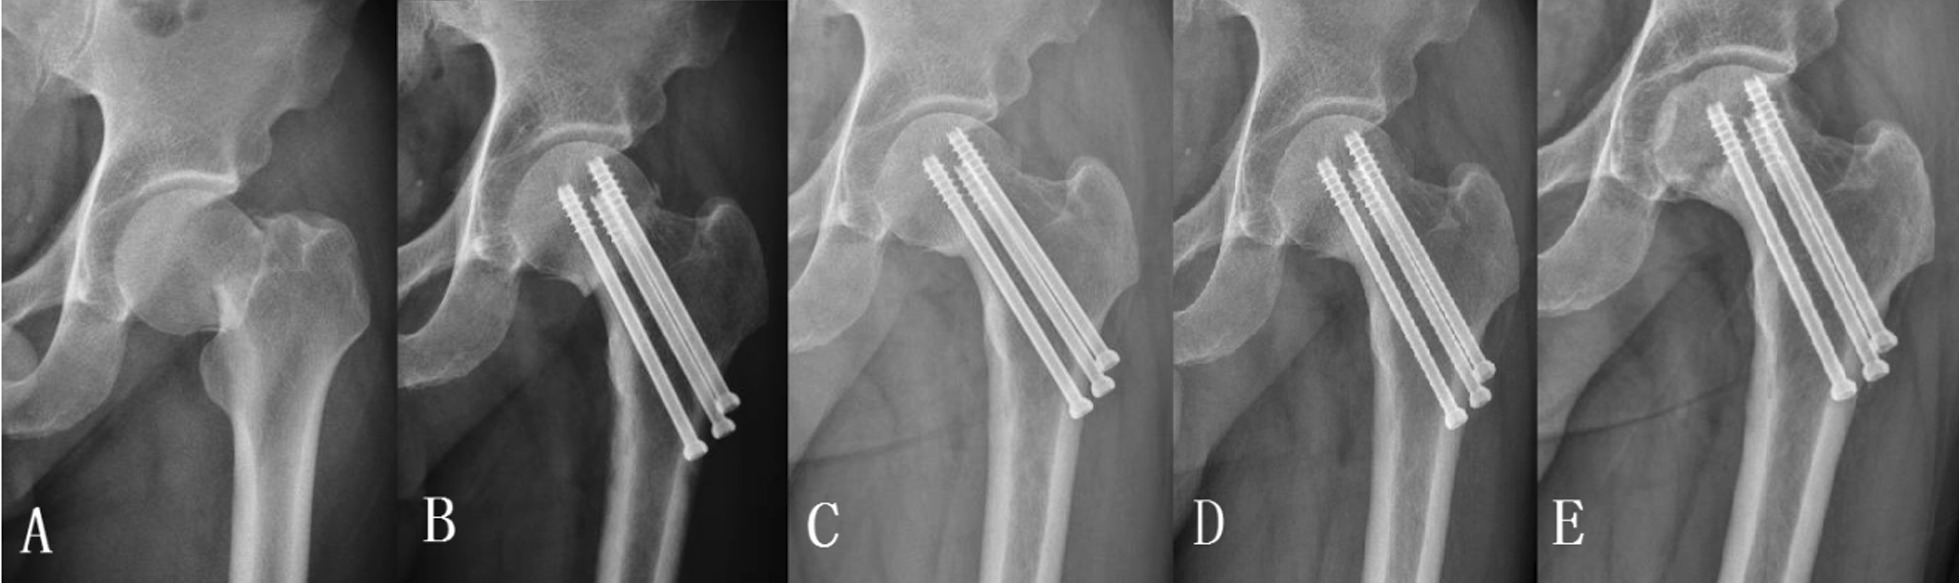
A 52-year-old man with left femoral neck fracture received ordinary cannulated screw fixation. The anteroposterior X-ray view of the proximal femur at pre-operation (A), 3 months (B), 9 months (C), and 2-(D) and 4-year (E) follow-up visits after operation. The left femoral neck fracture completely healed, but femoral head necrosis occurred
